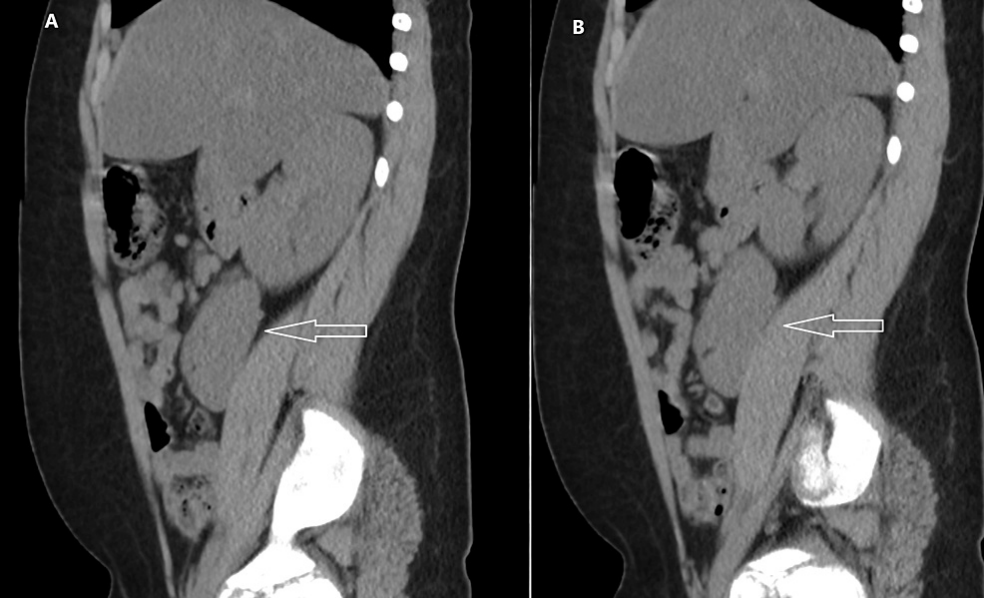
Sagittal CT images (A) and (B) demonstrate crossed renal ectopia (white arrow)
